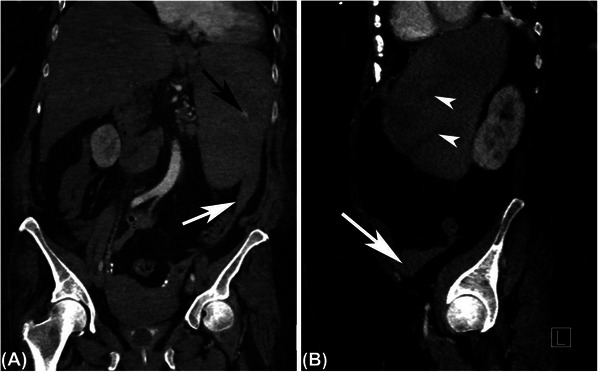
Contrast‐enhanced computed tomography. Coronal (A) and Sagittal (B) views of the abdomen and pelvis demonstrate a small area of contrast extravasation (black arrow) in an enlarged, heterogeneous spleen with multiple hypodense lines suggestive of lacerations (white arrowheads). Hemoperitoneum was also evident (white arrows).
